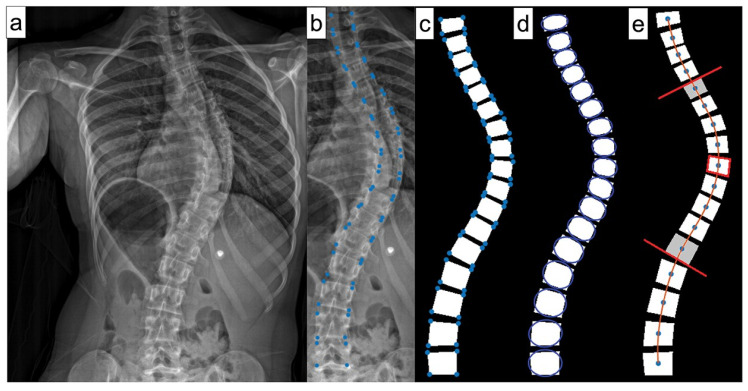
Steps of Cobb angle measurement for AI training from frontal radiographs. (a) Plain frontal X-ray. (b) Four landmark points (LMPs) were selected per vertebra. (c). Polygon fit through the LMPs of each vertebral vertex. (d) Best fit ellipse through each polygon to calculate the orientation of each vertebra as the angle between the major axis of the ellipse and the horizontal. (e) A cubic spline was fit through the centroids of the vertebrae, the most tilted vertebrae above and below the apical level were identified, and the Cobb angle was calculated between these vertebrae [15].
